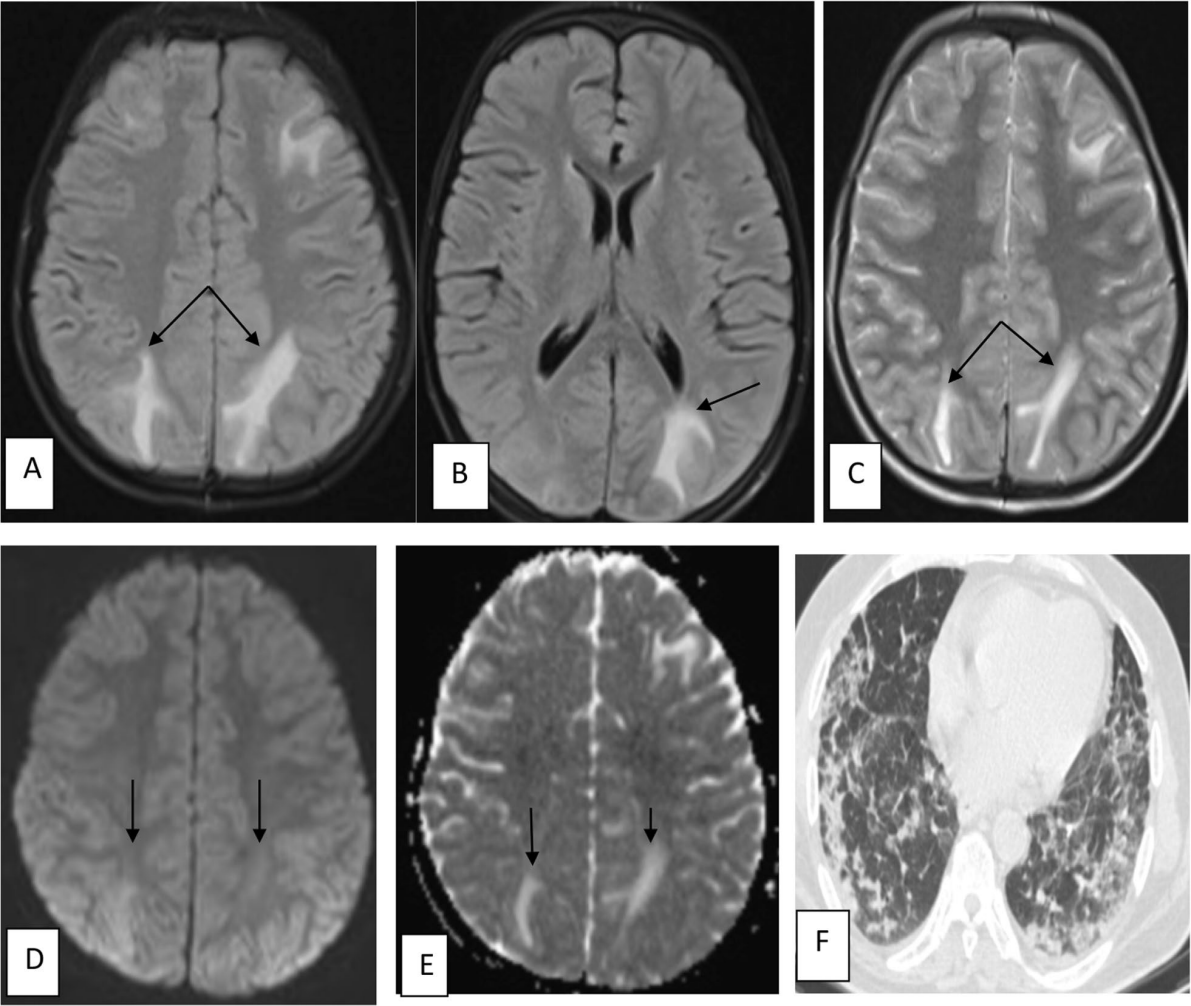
Female patient aged 40 years old known to be hypertensive with recent history of COVID-19 developed altered consciousness. MRI brain shows multiple nearly symmetrical subcortical patchy areas of altered signal intensity are seen at both high parietal and, occipital regions (black arrows) displaying high signal in FLAIR and T2 WI (A–C), free diffusion in DWI & ADC (D, E) this MRI features are suggestive of PRES (posterior reversible encephalopathy syndrome). Axial CT (F) chest cut of the same patient shows bilateral peripheral ground-glass opacities mixed with crazy-paving appearance (severe severity)
